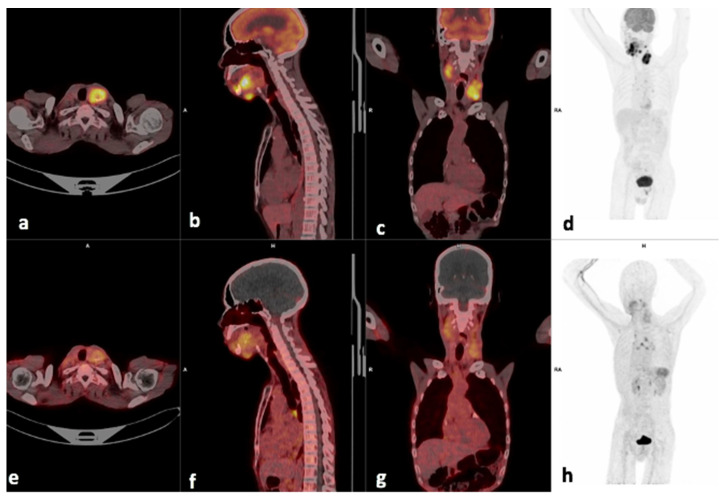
Example of a moderate CXCR4-positive, high FDG-positive patient. Display of [^18^F]F-FDG-PET/CT (a–d) and ^68^Ga]Pentixafor-PET (e–h) (interval between both scans, 14 days) in a 60-year-old male patient with oral cavity SCC (patient #6). [^68^Ga]Pentixafor-PET demonstrates less intense tracer accumulation at the site of primary SUVmax 4.84 (b) compared with 18.36 on [^18^F]F-FDG (f), the left cervical node metastasis also showed more intense uptake on [^18^F]F-FDG (c), SUVmax 14.41 versus 5.29 on [^68^Ga]Ga-Pentixafor (g).
